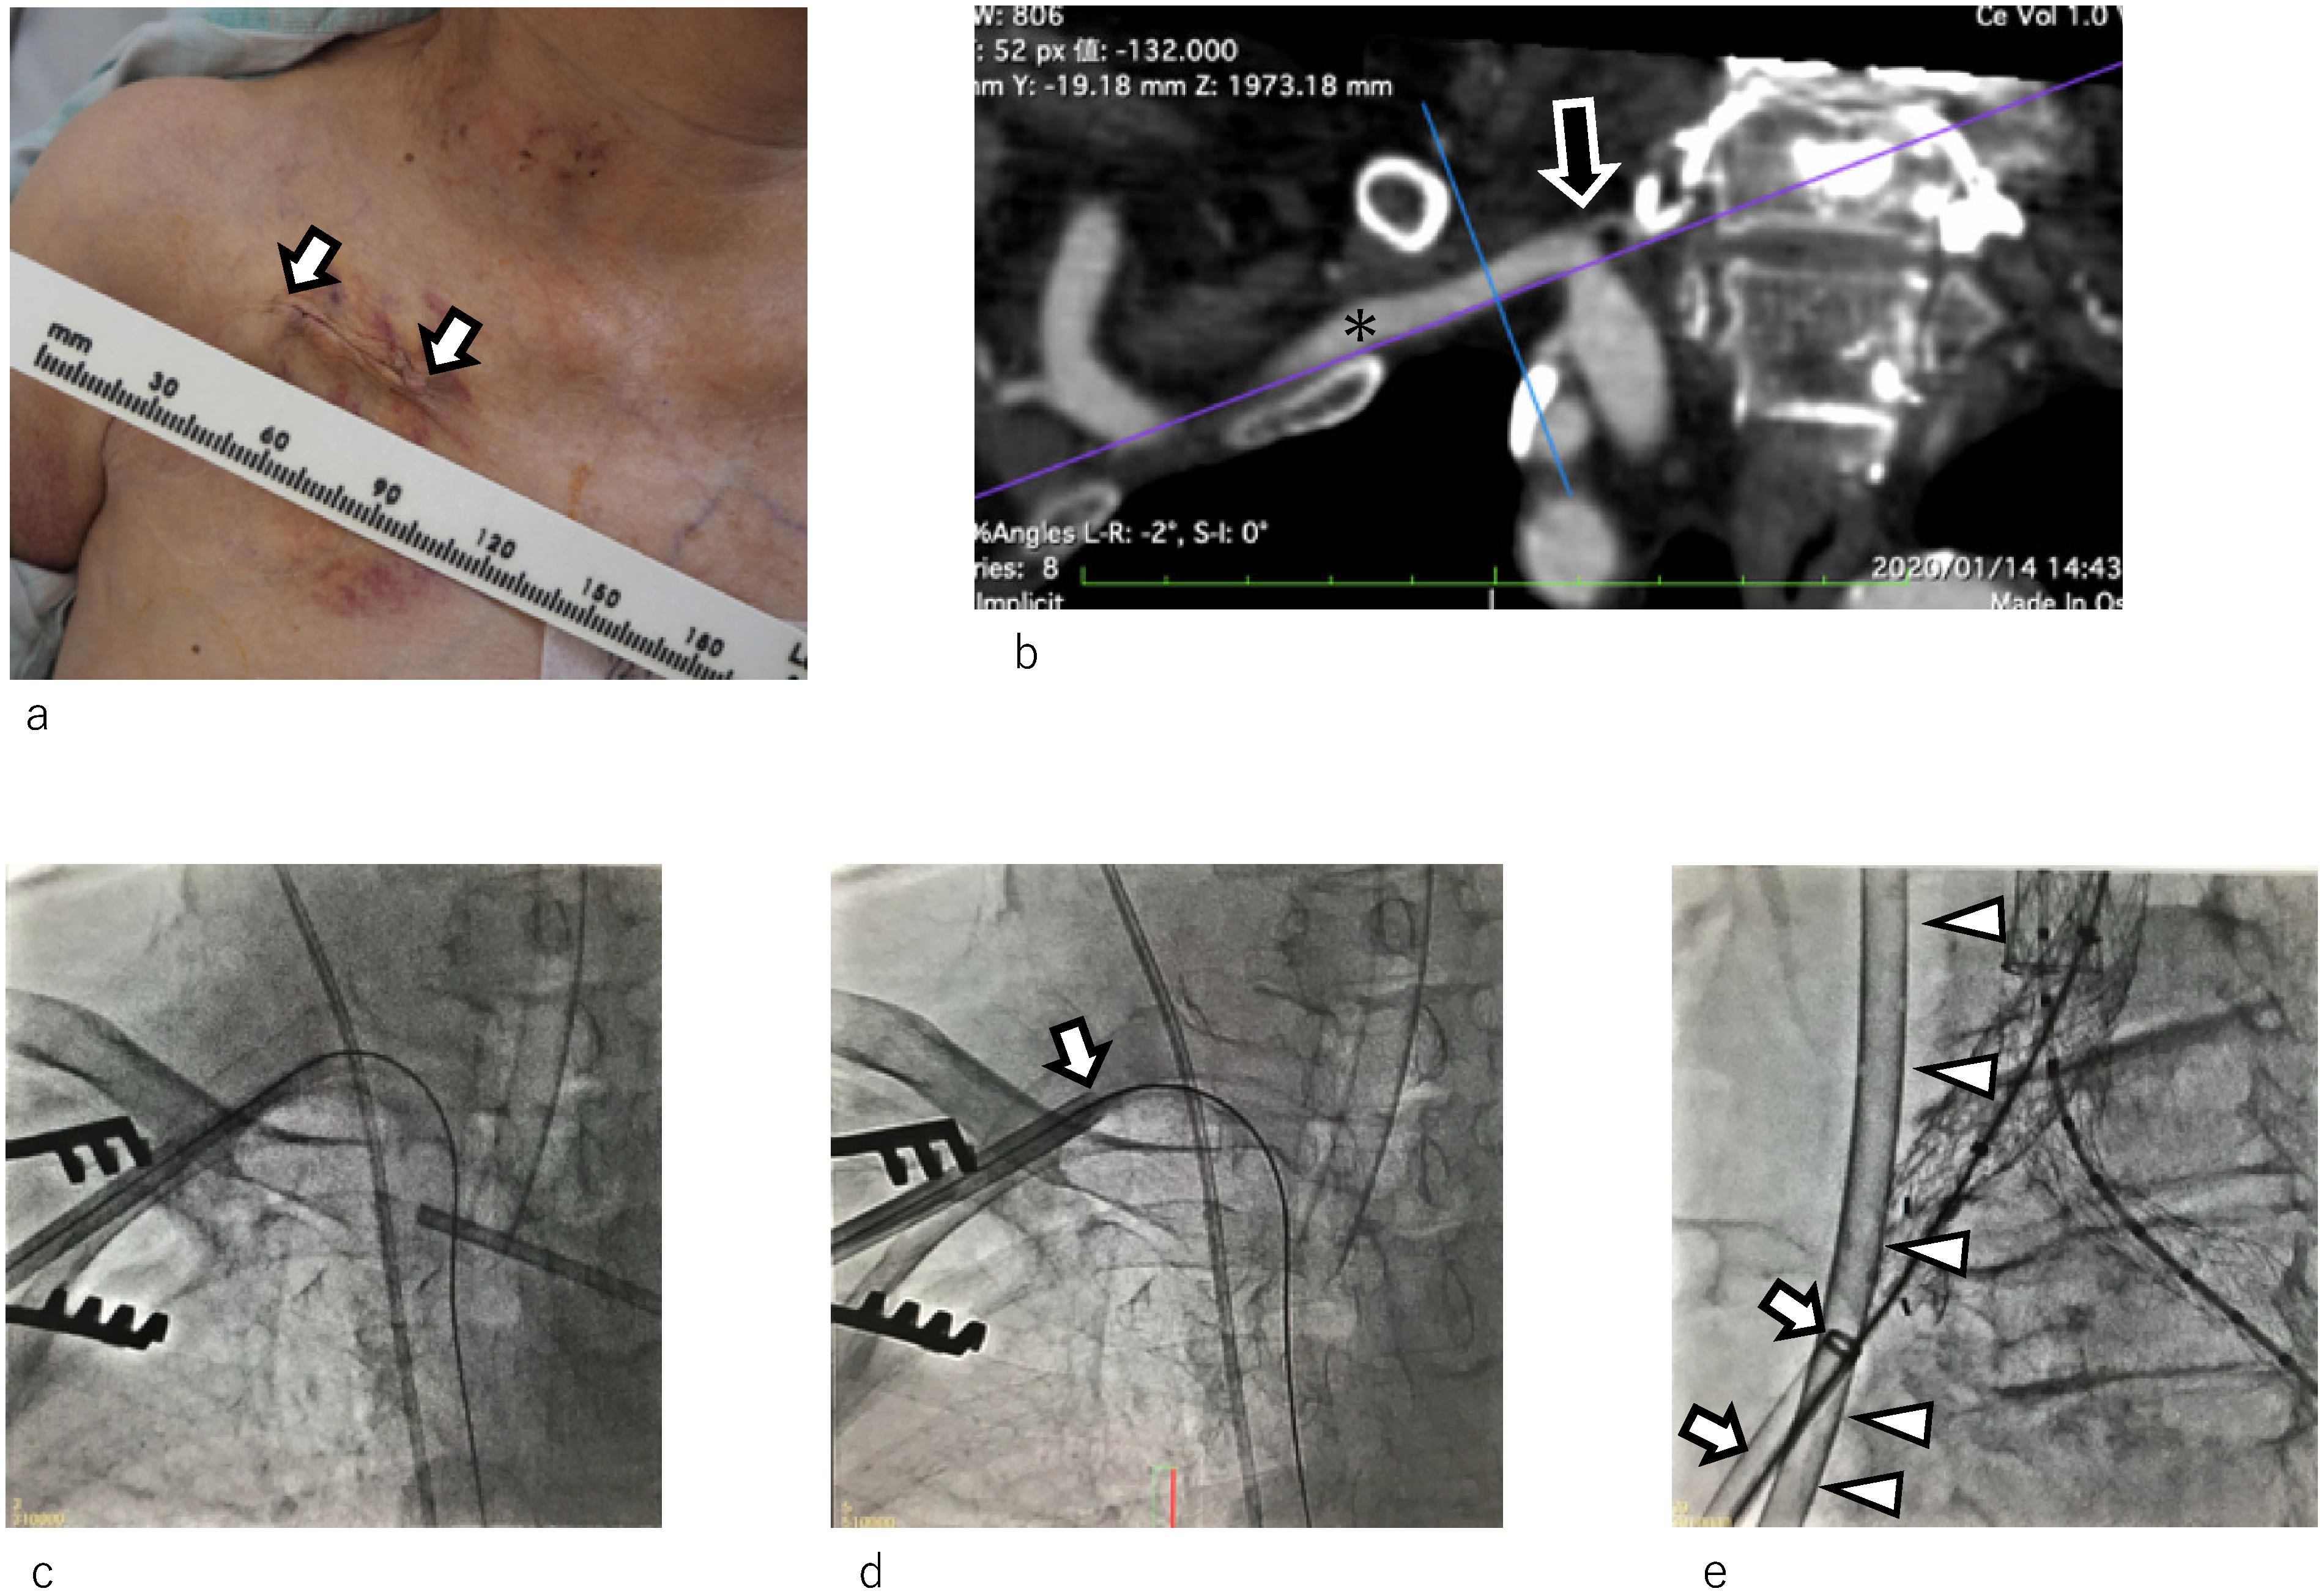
Fig. 2 Images during the surgery: (a) An image of the wound for the right axillary artery exposure and cannulation (arrows). (b) Preoperative computed tomography (CT) images of the right axillary and subclavian artery. An asterisk (*) denotes the planned cannulation site, and an arrow denotes the vertebral artery. (c) Fluoroscopy image during insertion of the inflow cannula. The cannula was inserted using the direct Seldinger method. (d) Fluoroscopy image after placement of the inflow cannula. (e) Fluoroscopy image during endovascular stent-graft placement. Arrow heads: 21 Fr outflow cannula, arrows: 16 Fr guiding sheath for stent-graft placement.
